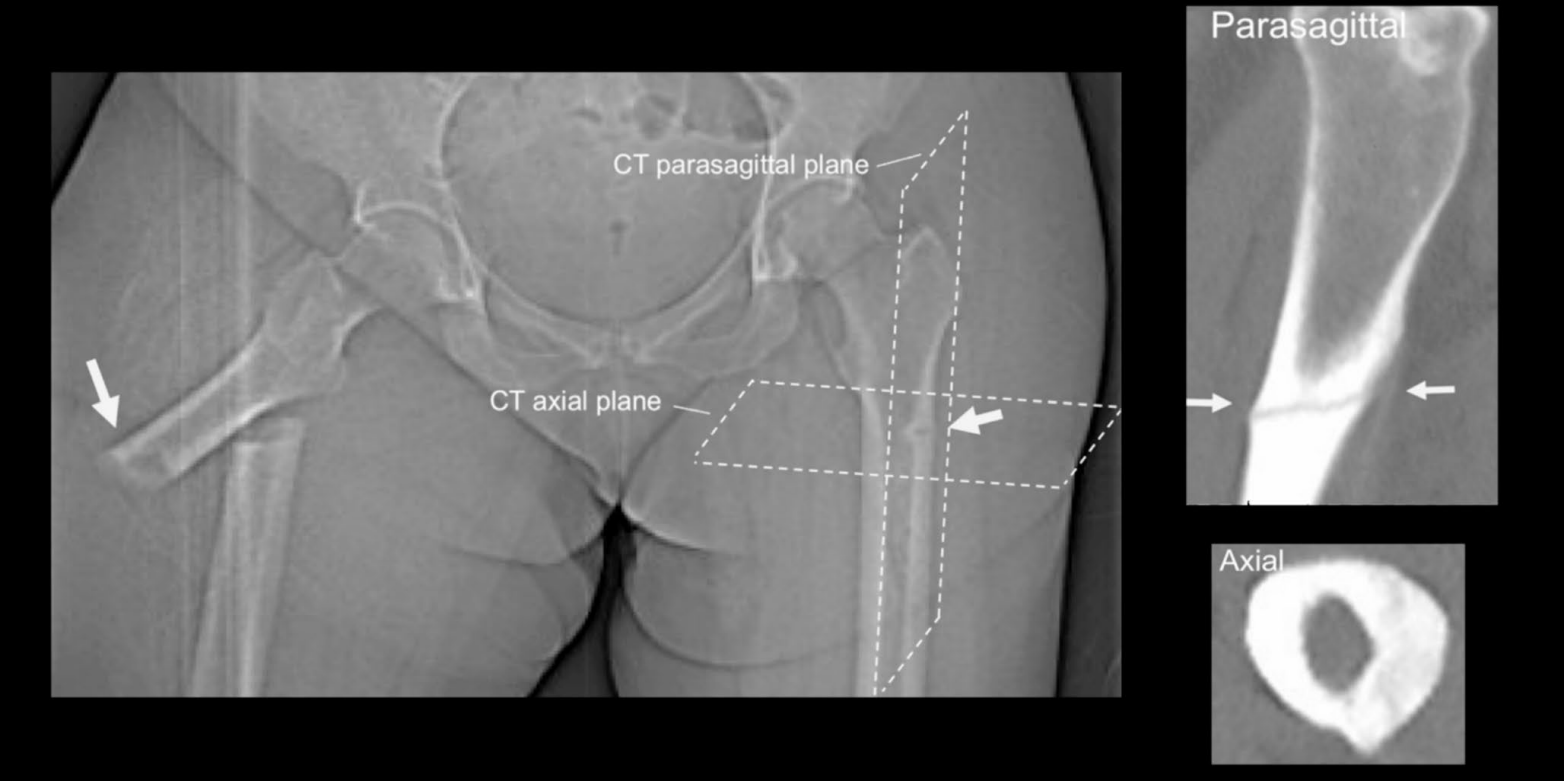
Classical lateral cortical bone fractures in HPP (a) CT scout view (left, main panel) of a 45-year-old female HPP patient whose first presentation was with a spontaneous ‘tensile’ fracture through her dense, thickened right femoral cortex. She was also found to have a left femoral endosteal thickening (arrowed). This endosteal expansion/callus seems widespread in this type of HPP fracture and is noted to be different to the periosteal ‘beaking’ of bisphosphonate-associated fractures. (b) Closer investigation of the left femoral cortex with oblique parasagittal (upper right) and axial (lower right) CT reconstructions showed an impending break with a large plateau of disconnected lateral femoral cortex. White arrows also show the extent of the macroscopically visible tensile fracture lines. Genetic analysis revealed compound heterozygosity at the ALPL gene (NM_000478:5: c.340G > A p.(Ala114Thr) and c.1363G > A p.(Gly455Ser))
